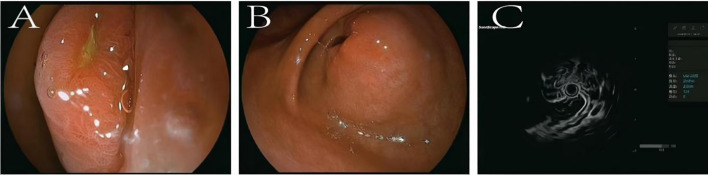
Endoscopic ultrasound and miniprobe ultrasound of the gastric antrum. (A) The mucosa exhibits alternating red and white areas, with the red areas predominating, and a 4 cm protrusion is visible. (B) A smooth surface with localized depression and ulceration, showing irregularities in the mucosal layer. (C) A suspected hypoechoic lesion originating from the muscularis propria, with unclear boundaries due to the limited scanning range of the miniprobe ultrasound.
